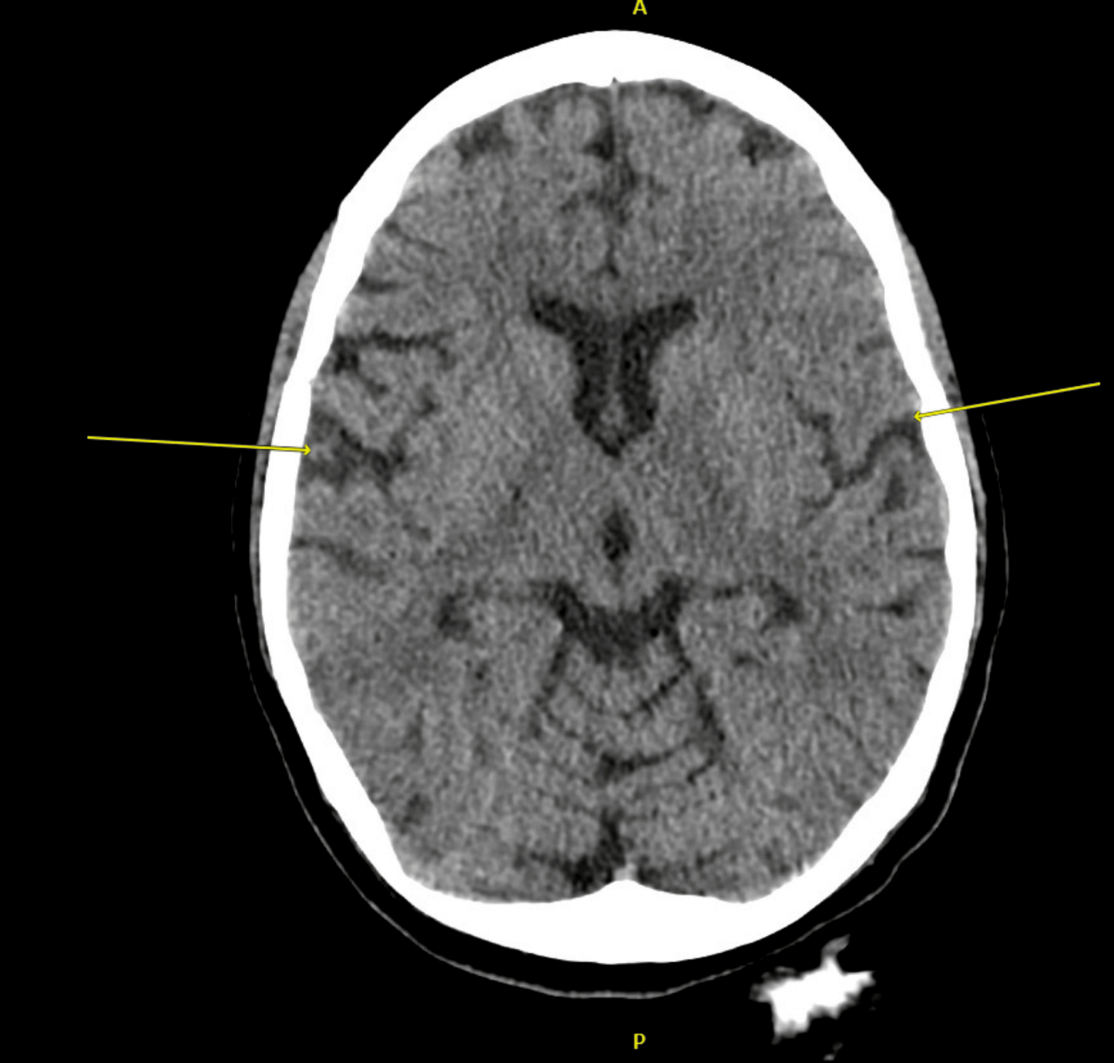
CT scan of the brain without contrast, showing mild enlargement of the lateral ventricle and diffuse cortical atrophy, characterized by mild widening of lateral fissure (yellow arrows)
